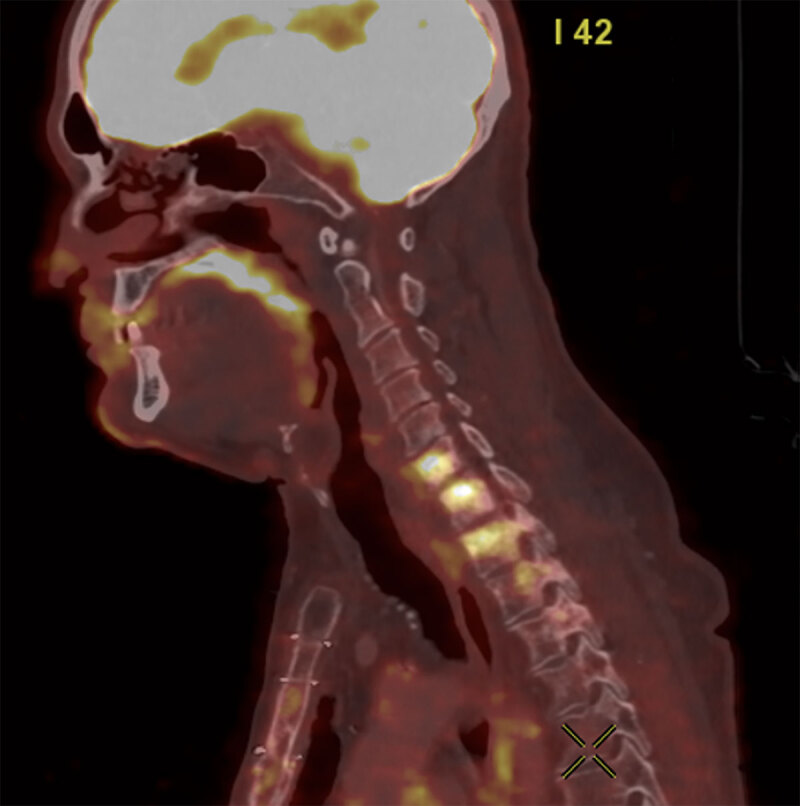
Spine magnetic resonance imaging (MRI) performed two days later didn’t reveal any vertebral signal abnormalities (Figure 5). Nor were there any bone condensing metastatic lesions depicted on a non-enhanced CT scan of the cervical spine performed after the MRI (Figure 6).
